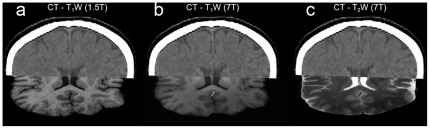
Registration of 7T MRI to CT. An example of co-registration between CT and a) 1.5 T T_1_W, b) 7 T T_1_W, c) 7 T T_2_W. Although CT images are far less informative than MRI, it can be seen that the ventricles are perfectly aligned, indicating an adequate quality of registration. Registering 1.5 T MR images to a CT is a common practice for planning of neurosurgical procedures including DBS surgery and tumor resections. This practice allows for using the superior contrast of MRI while capitalizing on the geometric integrity of the CT image. Here we suggest that the same practice may be used for correcting for whatever geometrical distortions that may be present in 7 T MRI.
